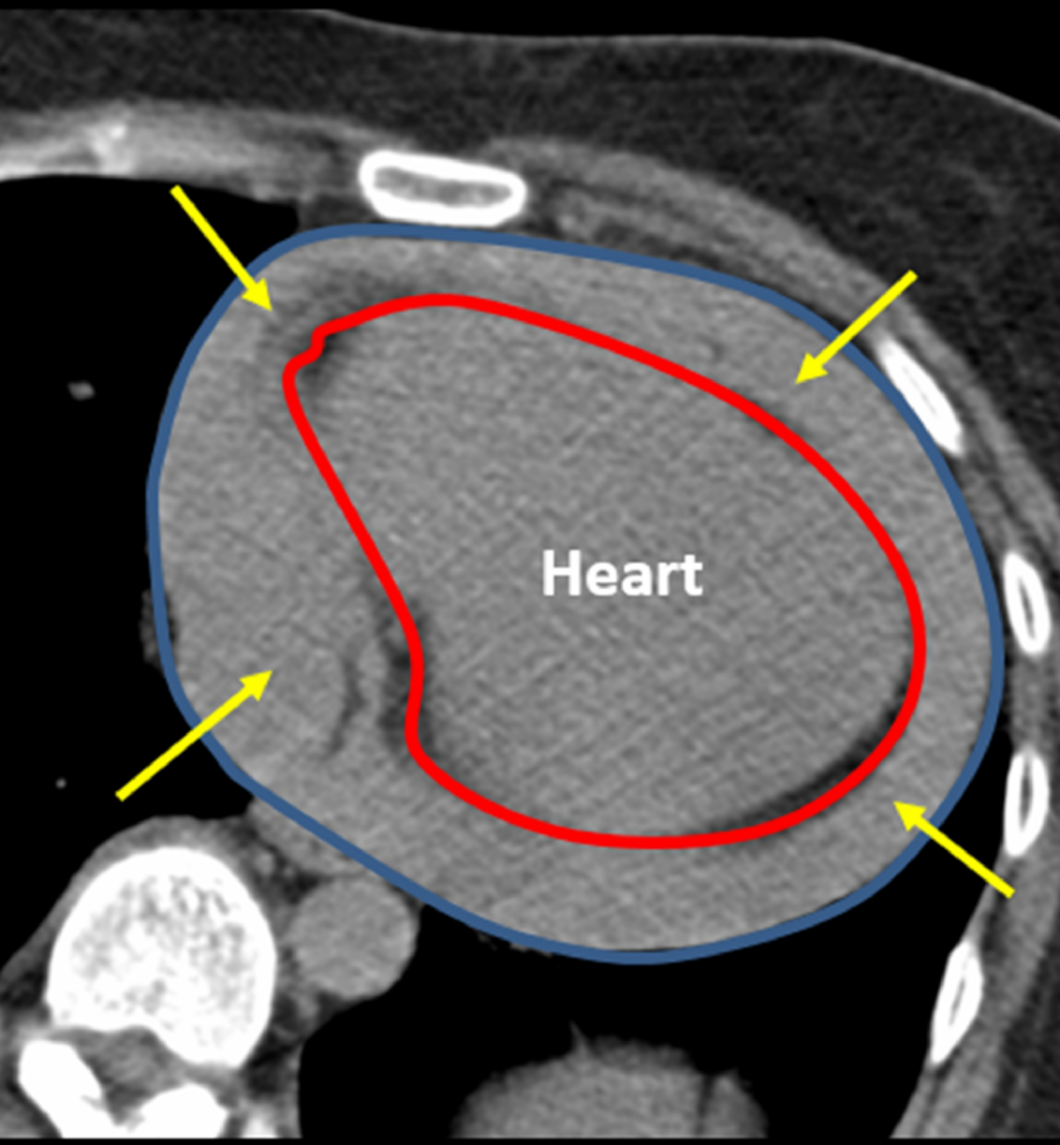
Post-biopsy Acute Cardiac Tamponade A computed tomography of the axial chest after taking several samples of the anterior mediastinal mass shows acute cardiac tamponade. The blood collection can be visualized within the pericardial space (yellow arrows), between the heart (red outline) and the pericardium (blue outline).
